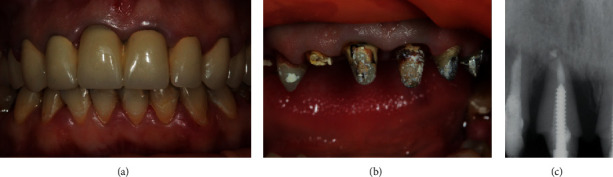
(a) Frontal area of the upper jaw. (b) After removal of metal-ceramic crowns. (c) Periapical X-ray of the tooth 11.
